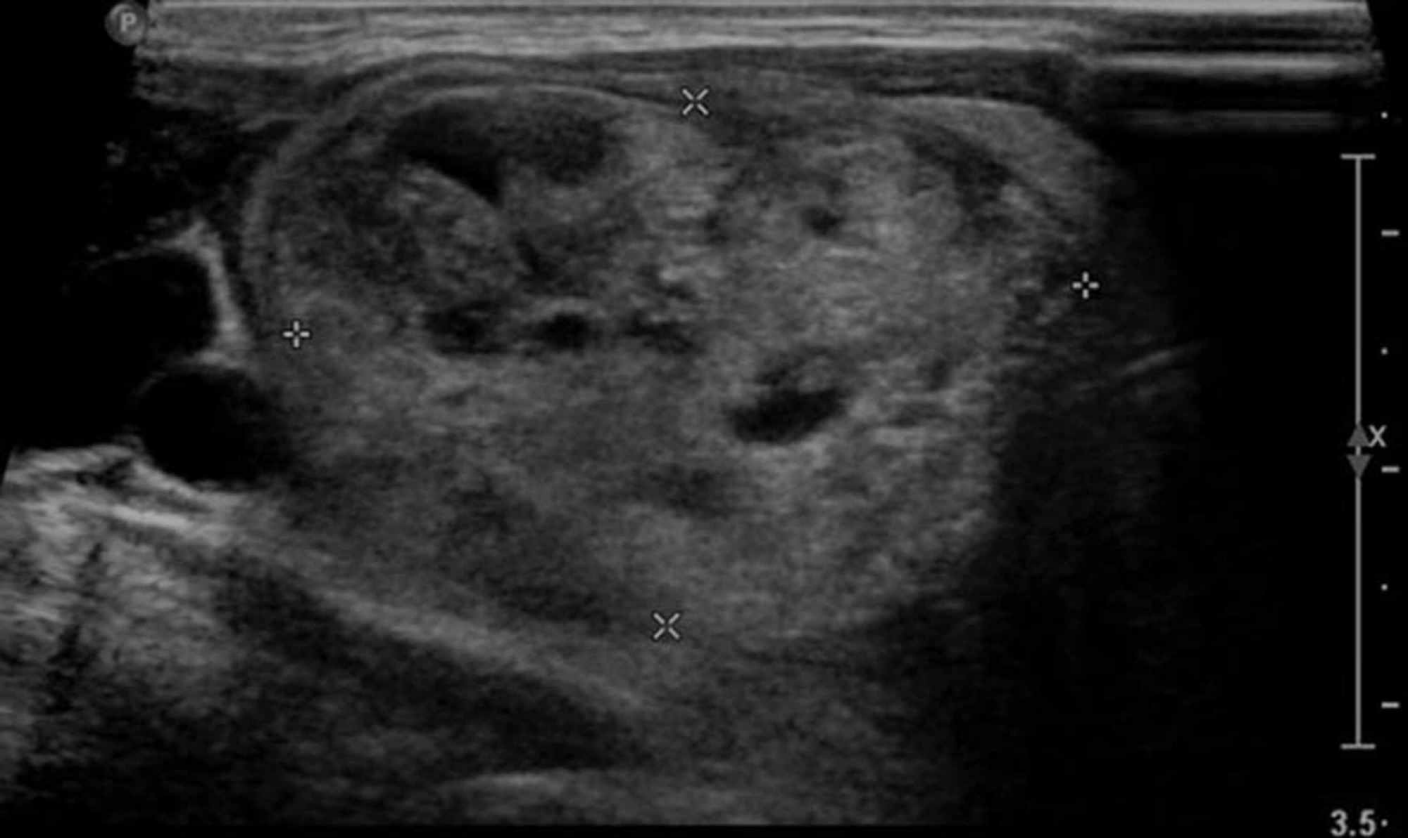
U/S of neck multinodular goiter in the right side measuring 3.2 x 2.4 cm U/S, ultrasound.
